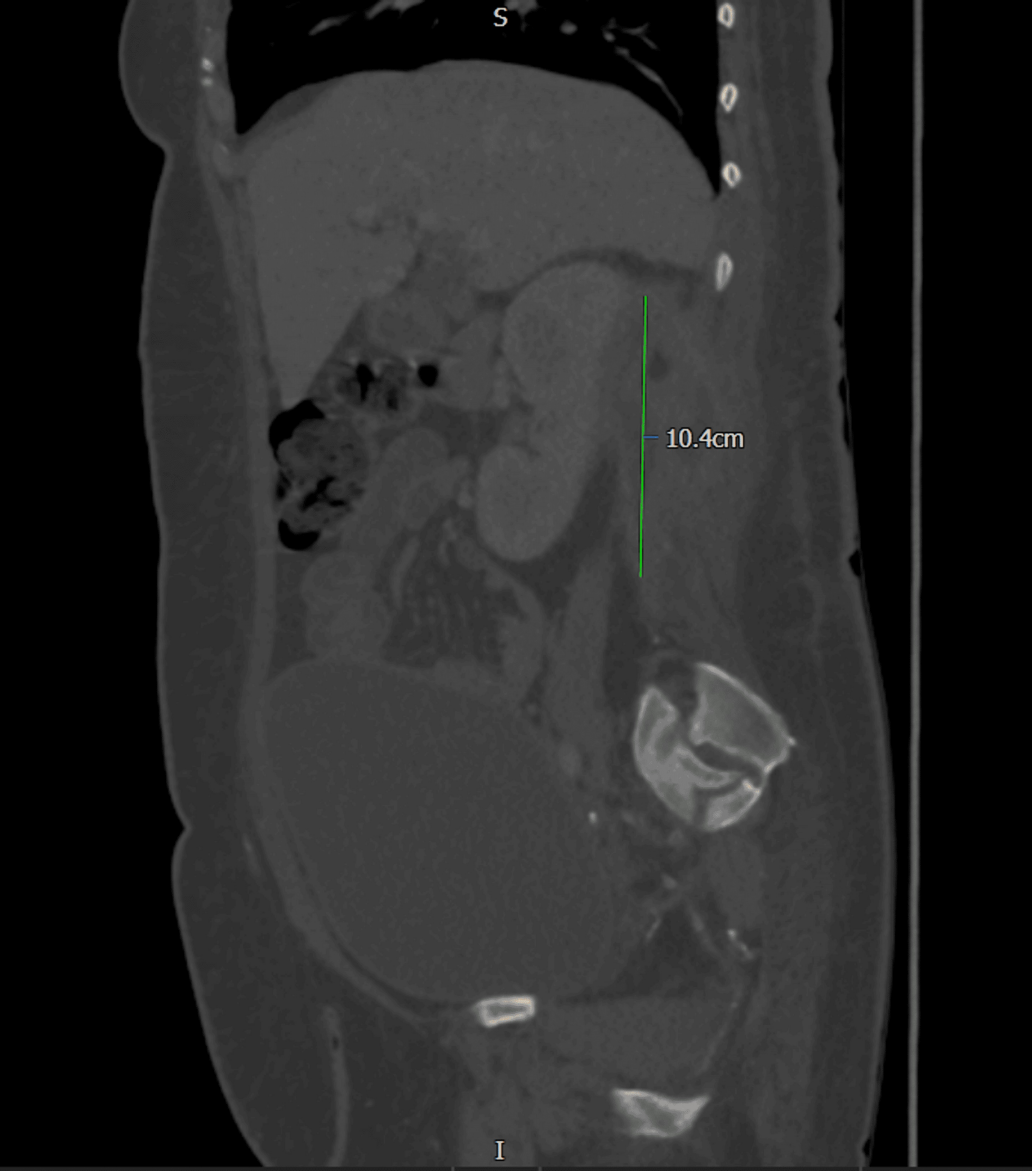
CT abdomen pelvis with contrast done on week 3 of admission shows Rt perinephric collection to be collapsed than before. It has an irregular appearance with multiple ramifications in the soft tissue. The abscess is 3.6 cm x 2 cm in dimension; however, there are soft tissue inflammatory changes surrounding the abscess measuring 10.4 cm x 5.2 cm. This makes the exact measurement of the abscess difficult CT: computed tomography
